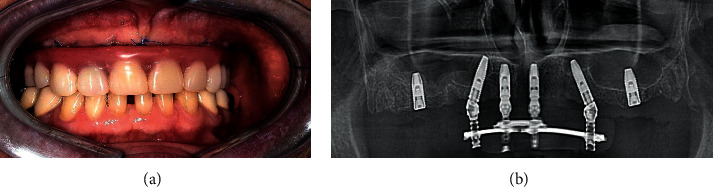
Immediate loading on the day of surgery using multiunit system and radiographic control.
